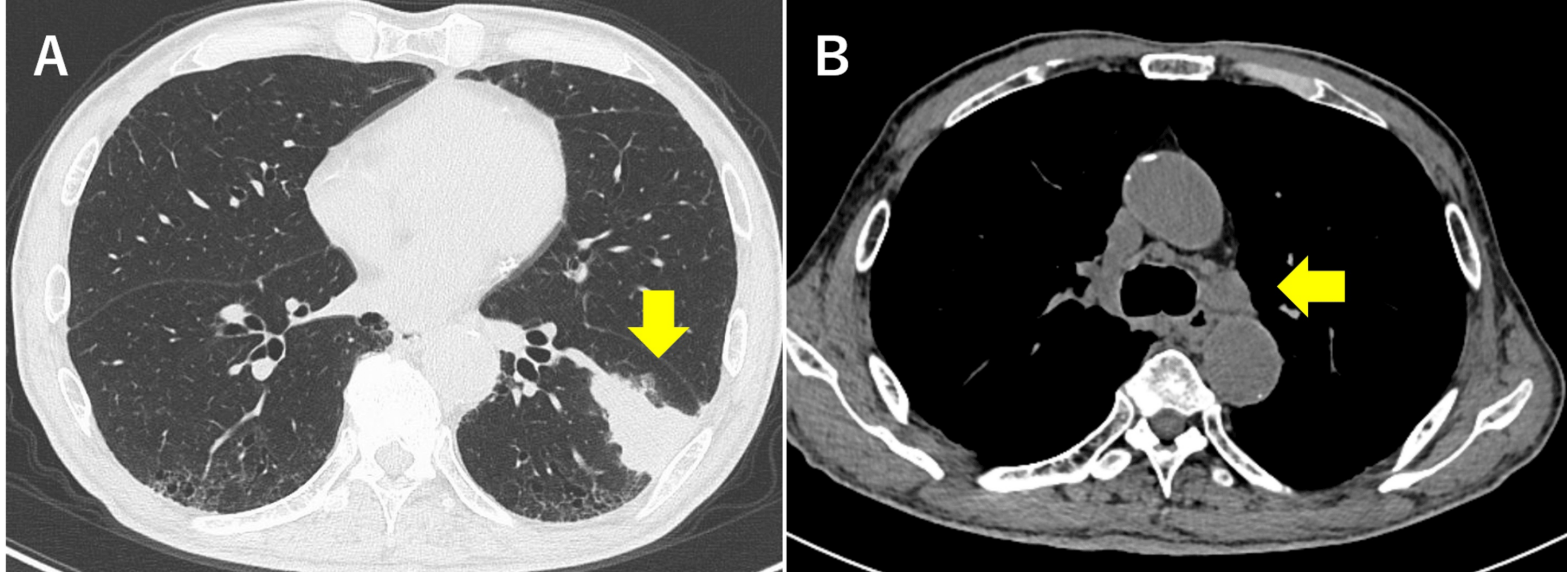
The tumor in the left lower lobe. (A) Computed tomography revealed a tumor (arrow) measuring 40×45 mm in the left lower lobe. (B) The #10 left (L), #11, and #12L lymph nodes were enlarged consecutively (arrow).
